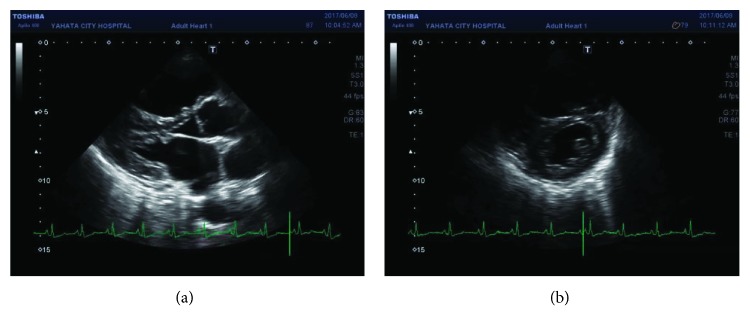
Transthoracic echocardiography shows that the left ventricle was compressed due to the enlargement of the right ventricle during end-diastole in deteriorating pulmonary hypertension, parasternal long-axis view (a), and parasternal short axis view (b).
